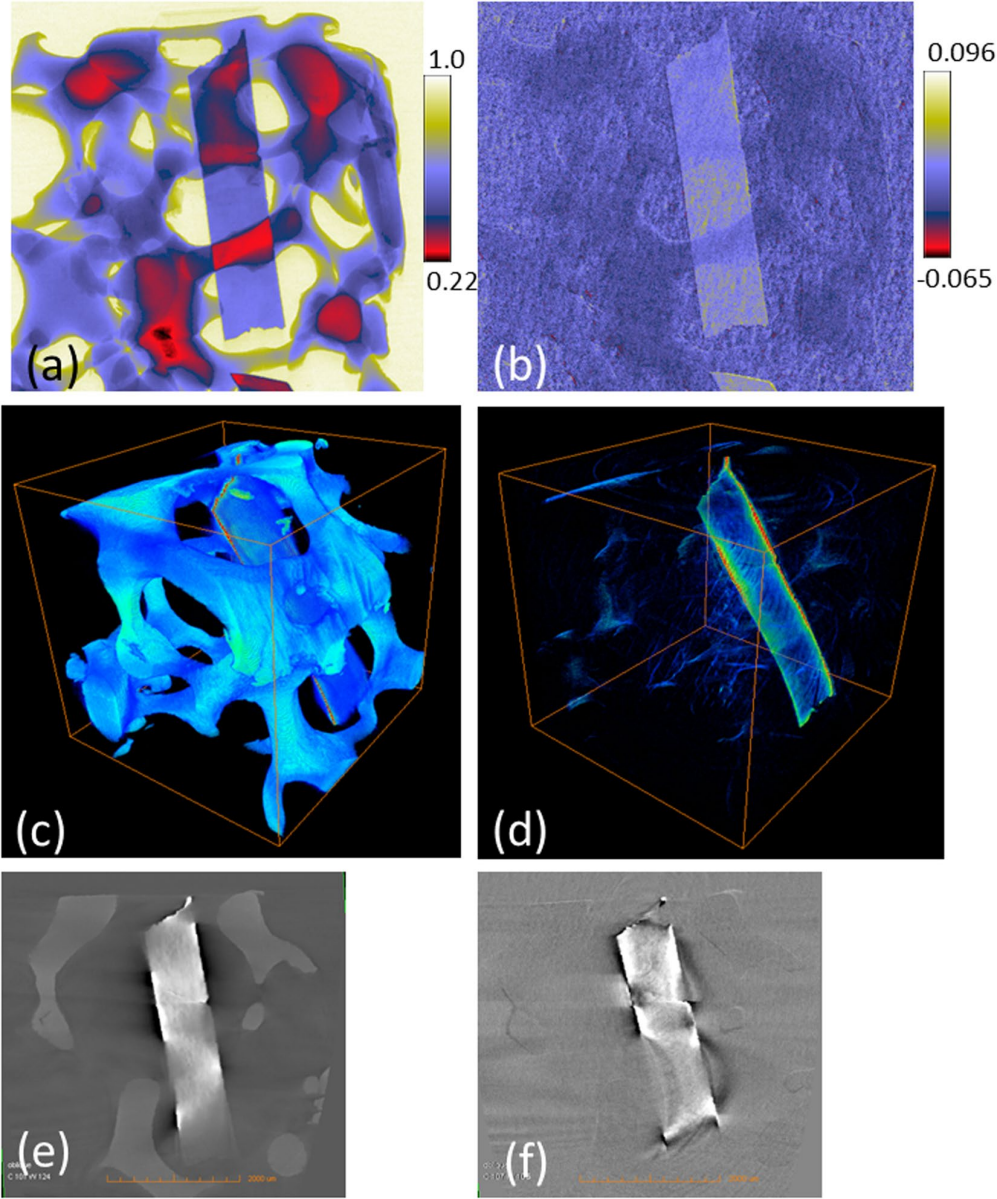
Three-dimensional elemental contrast demonstration. (a) and (b) shows one projection image before and after elemental contrast enhancement. Three-dimensional reconstructed image without applying elemental contrast is shown in (c) with the corresponding CT slice in (e). The elemental contrast method applied to each projection is shown in (d) as a three-dimensional reconstructed image of Rh material with the corresponding slice in (f).
